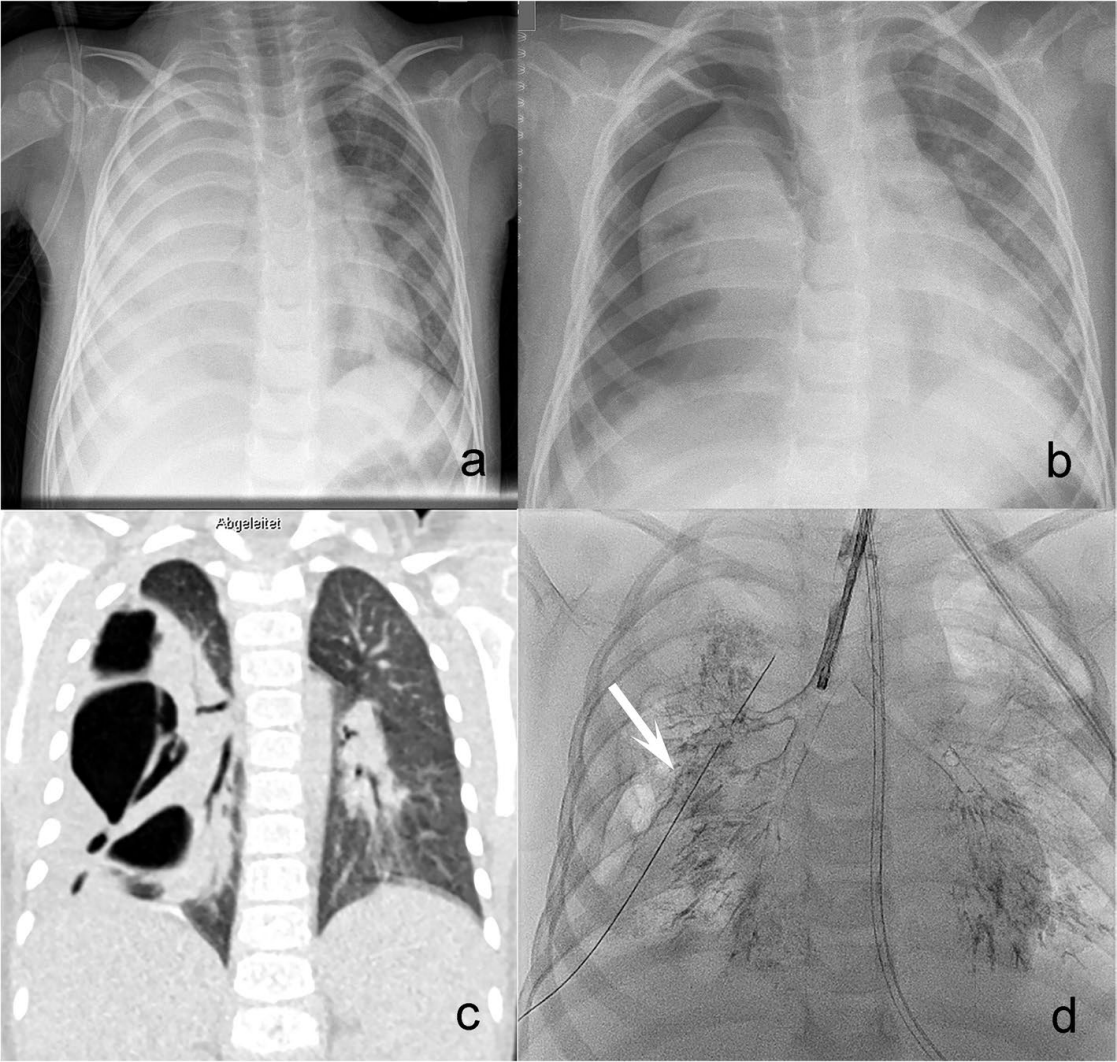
a Chest Xray with right sided pneumonia and effusion. b Chest Xray with tension pneumothorax right sided. c CT-Scan, coronary slice, pneumonia with dystelectasis, lateral pneumothorax and right sided fistula. d Bronchography with proof of PAL bronchus 3r (white arrow)
